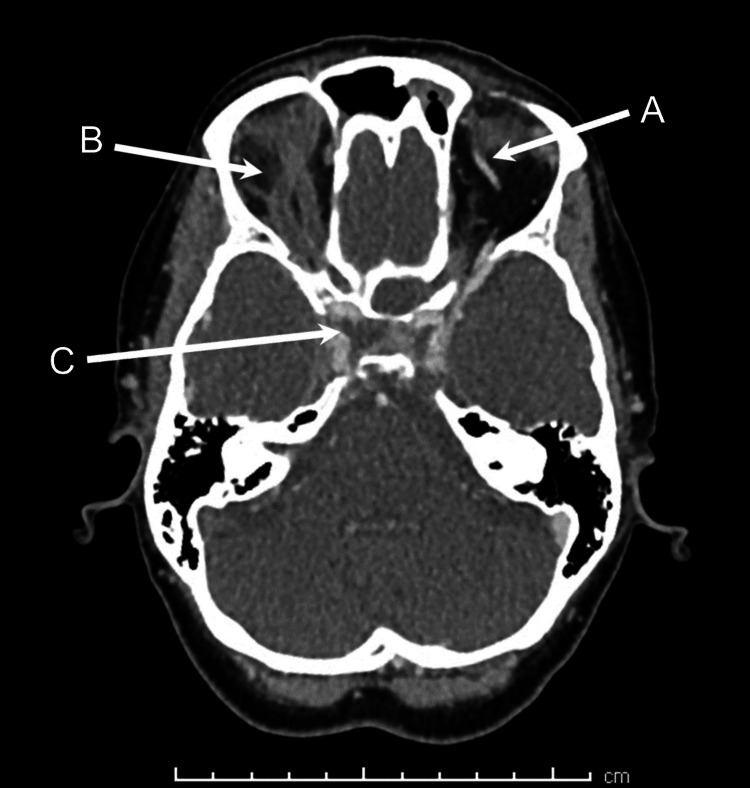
CT image showing the normal left ophthalmic vein (A), thrombosed right ophthalmic vein with retro-orbital fat stranding (B), and filling defect indicating right CST (C) CST, cavernous sinus thrombosis
